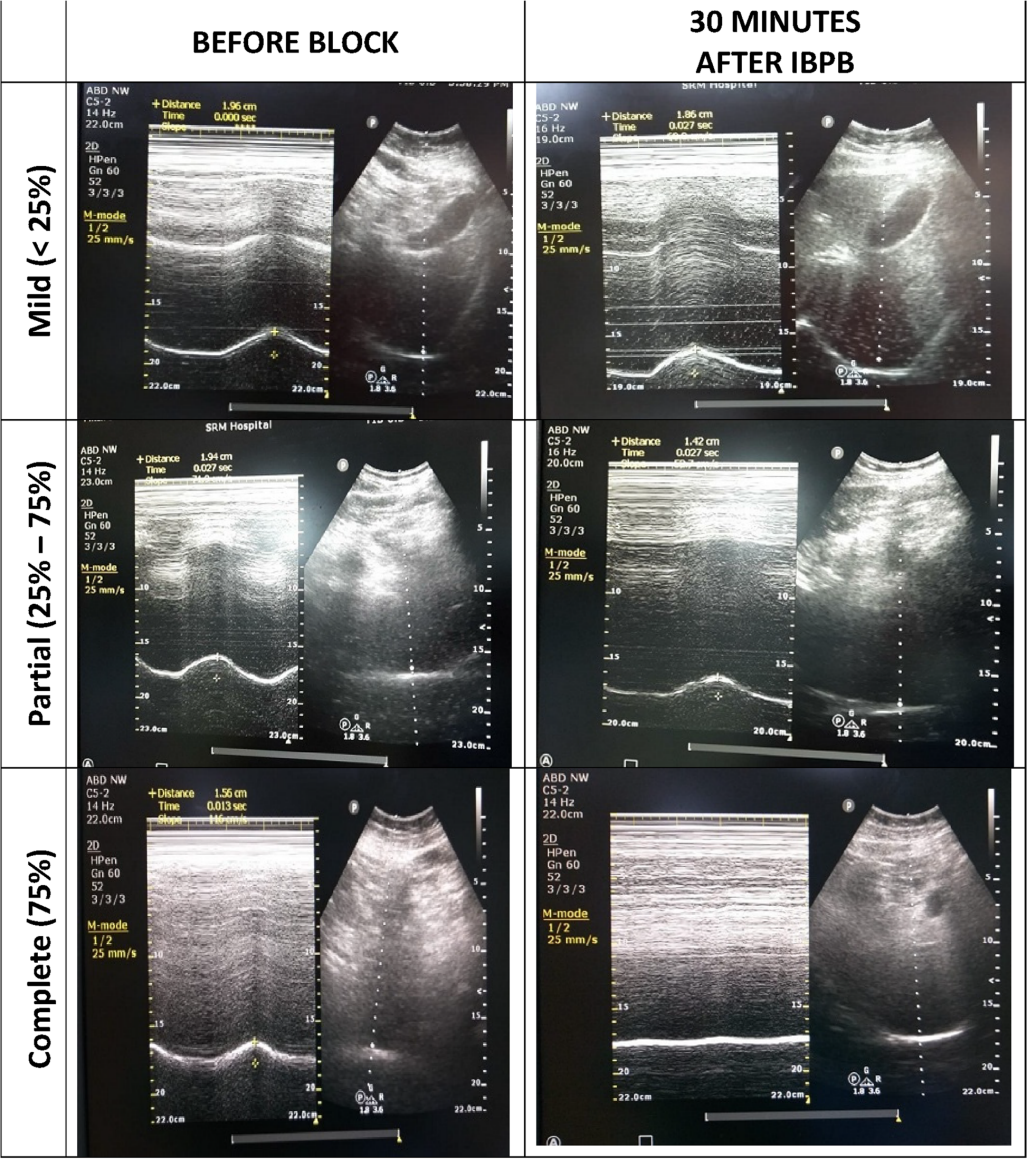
Ultrasound image before and after the IBPB and different degrees of diaphragmatic paresis
